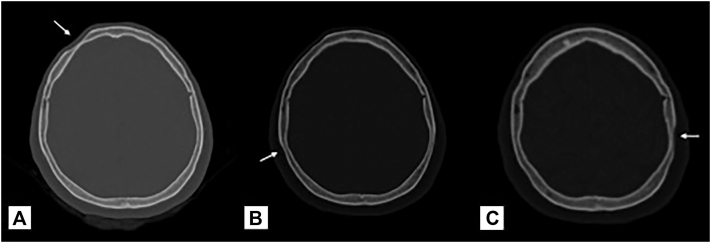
Axial brain CT demonstrating multifocal calvarial thinning. A, Discrete, noncontiguous right frontal calvarial thinning with near obliteration of the diploic space and inward contouring (*arrow*). B, Subtle shallow depression of the right parietal calvarium with some diploic narrowing (*arrow*). C, Focal inward depression of the left parietal calvarium with moderate to severe diploic space narrowing (*arrow*). *CT*, Computed tomography.
